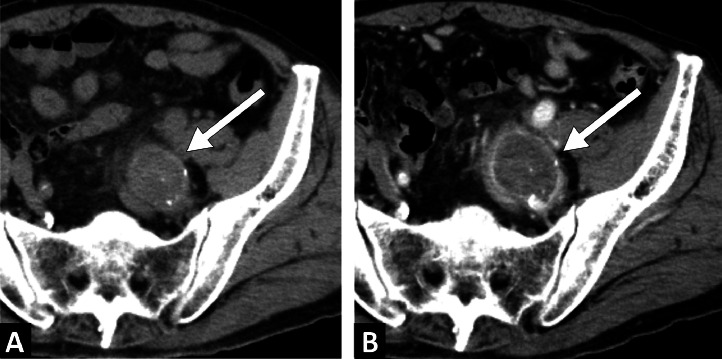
(A) Computed tomography initially conducted at another hospital showing a left internal iliac artery aneurysm with a maximum short diameter of 30 mm (arrow). (B) Computed tomography angiography conducted one week after the initial scan showing the left internal iliac artery aneurysm rapidly enlarged to a maximum short diameter of 38 mm (arrow).
